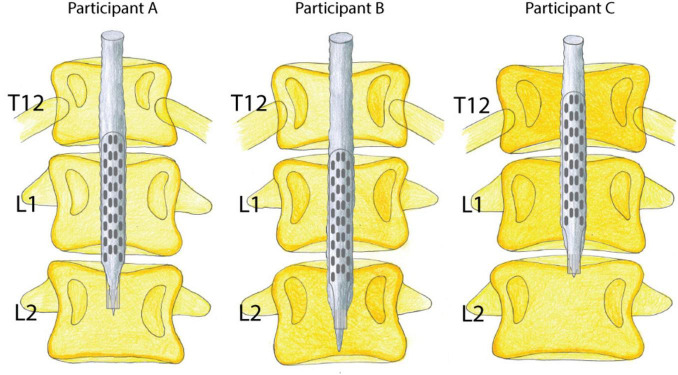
Visualization of paddle electrode placement in relation to the conus medullaris and vertebral bodies. Lumbosacral spinal cord reconstruction from MRI scans and X-rays; estimated epidural stimulation paddle placement in all three participants.
